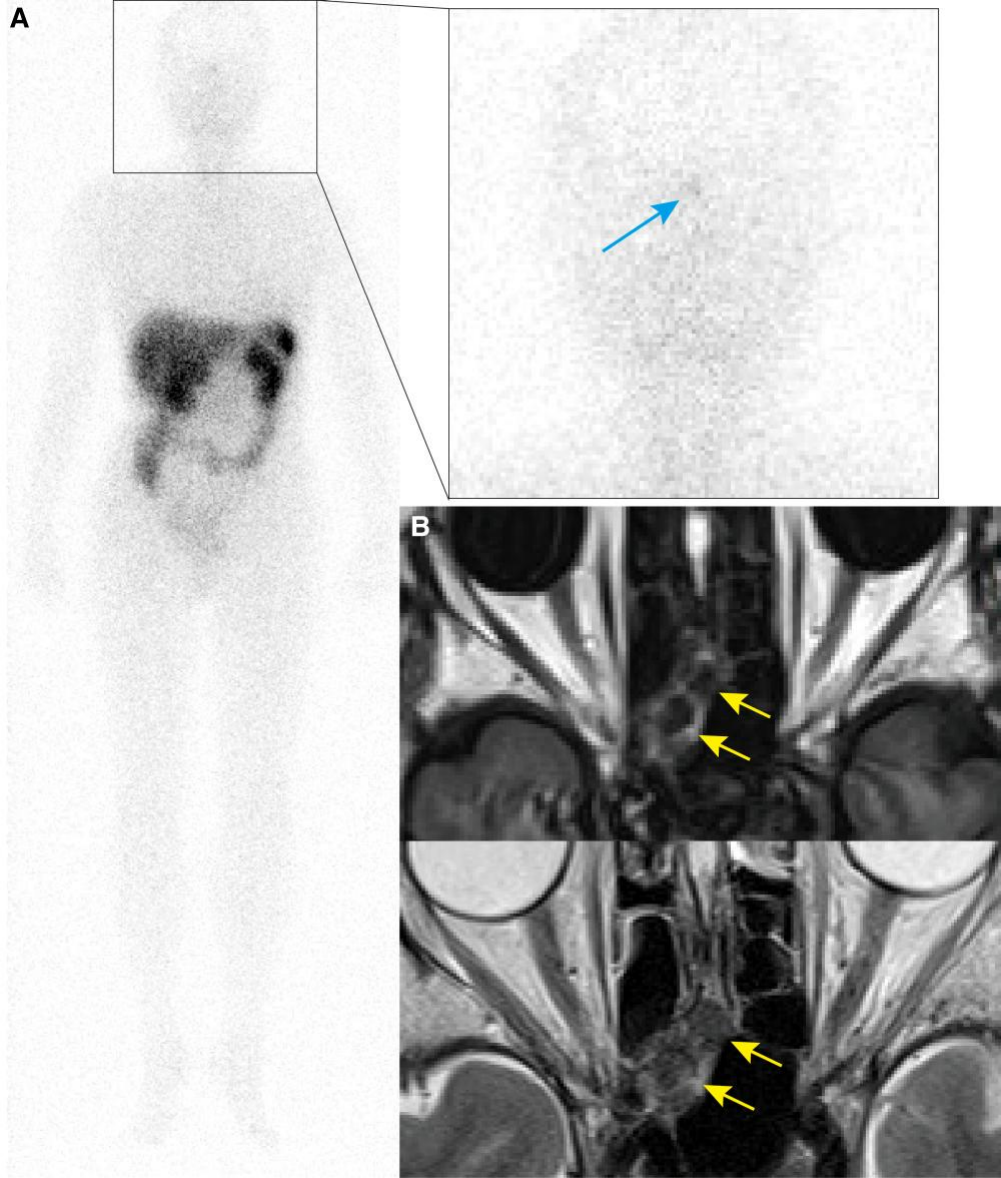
^111^In-pentetreotide scintigraphy and MR imaging. A, ^111^In-pentetreotide scintigraphy: A subtle uptake in the midline of the head (blue arrow). B, MR imaging: Mass-like lesions at posterior ethmoidal sinus with low signal intensity in both T1- and T2- weighted images (yellow arrows).
